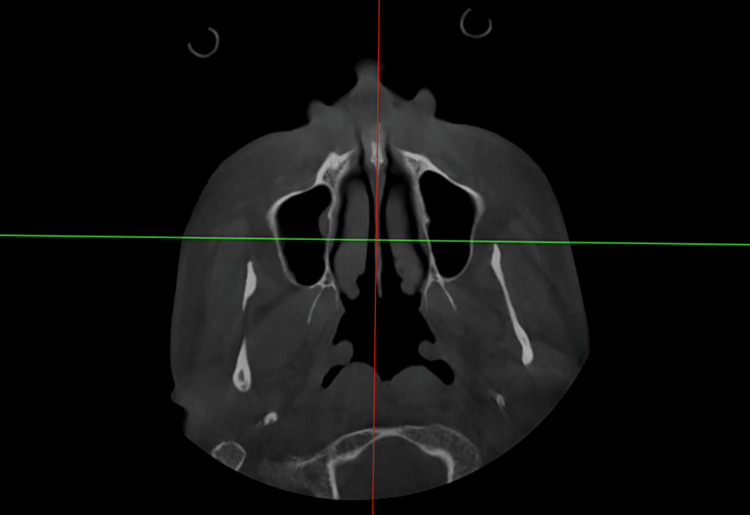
Midsagittal plane passing through the skeletal landmark crista galli in the CBCT CBCT: Cone Beam CT
